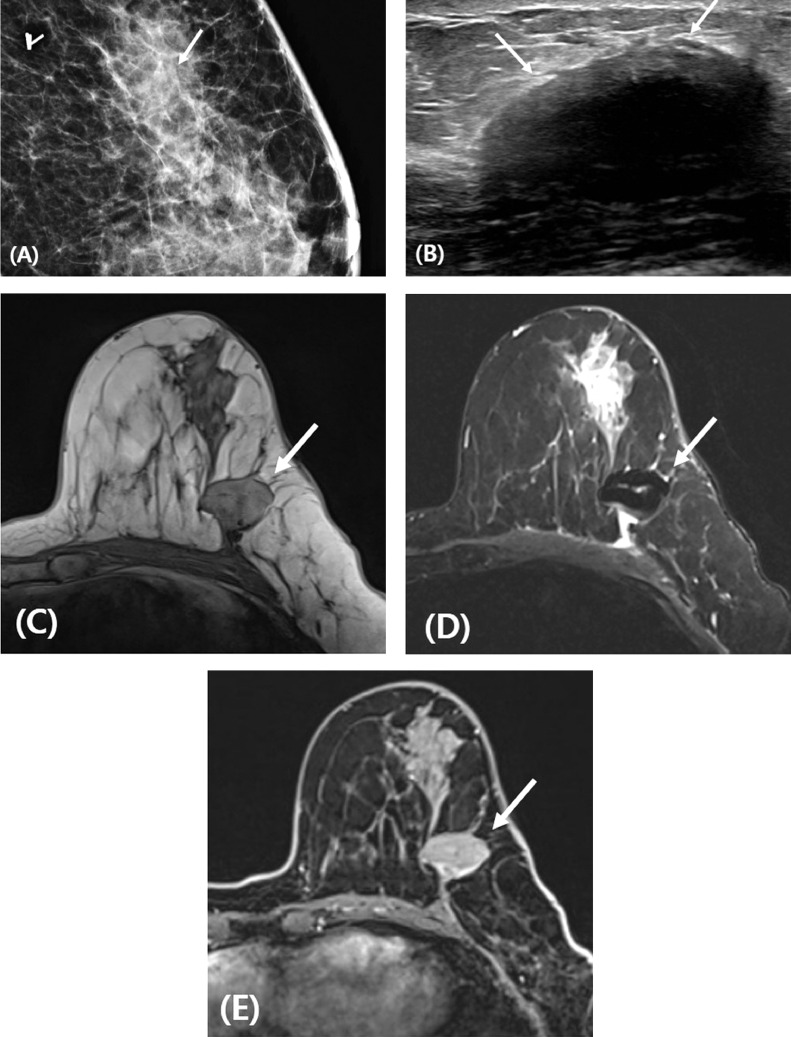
Volume replacement with an acellular dermal matrix (ADM) after right breast-conserving surgery. (A) The ADM is an oval shaped, circumscribed, isodense mass (arrow) on mammogram. (B) The ADM is a hypoechoic mass with posterior acoustic shadowing (arrows) on ultrasound. (C-E) MR images show the ADM (arrows) with iso-signal intensity on the T1-weighted image (C), low signal intensity on the fat-suppressed T2-weighted image (D), and no enhancement on the contrast-enhanced, fat-suppressed, T1-weighted image (E).
